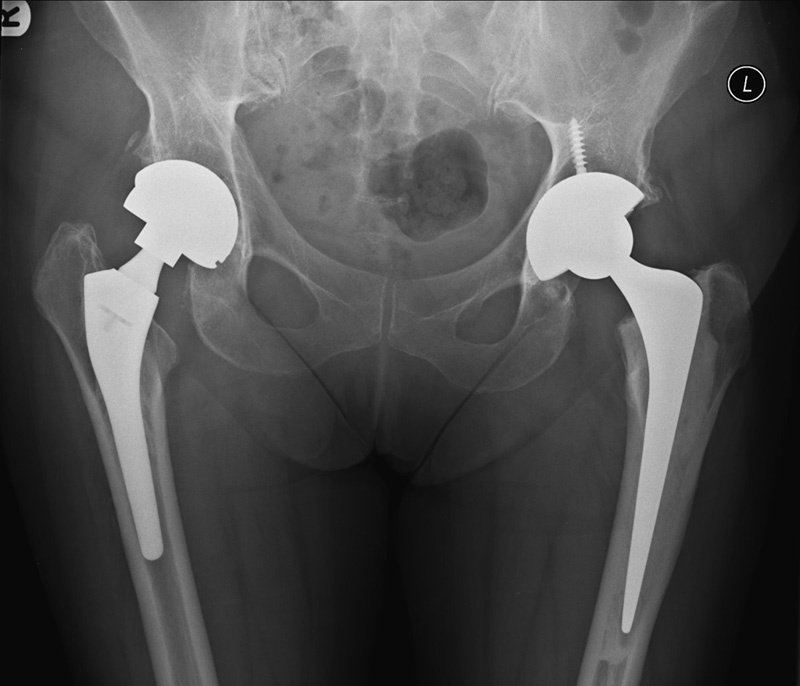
Anteroposterior radiograph demonstrating bilateral total hip arthroplasties with no adverse features.
